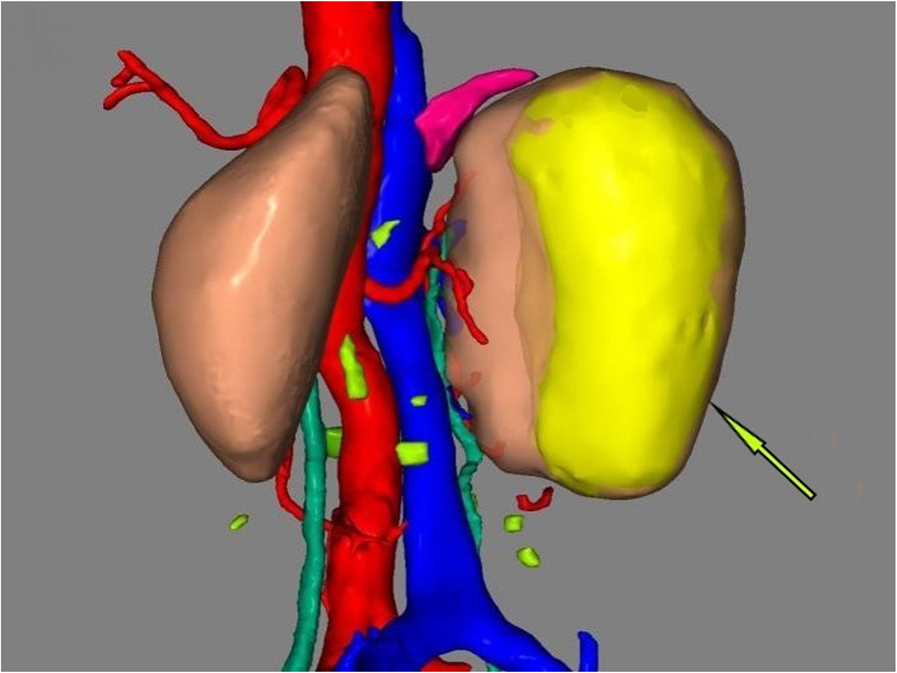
Spiral CT 3D image showing the annular calcification wall around the right kidney, with a size of 11.5 cm * 6.9 cm * 5.1 cm
